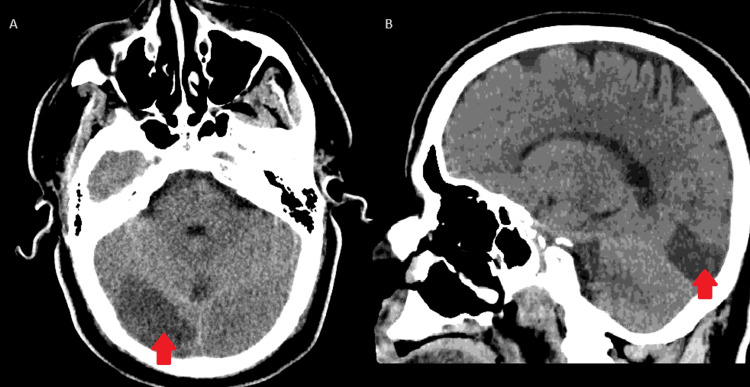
CT head with axial (A) and sagittal (B) views showing no evidence of hemorrhage but notable for a hypodensity in the right occipital lobe (red arrow), indicating the possibility of a developing ischemic infarct in the region
